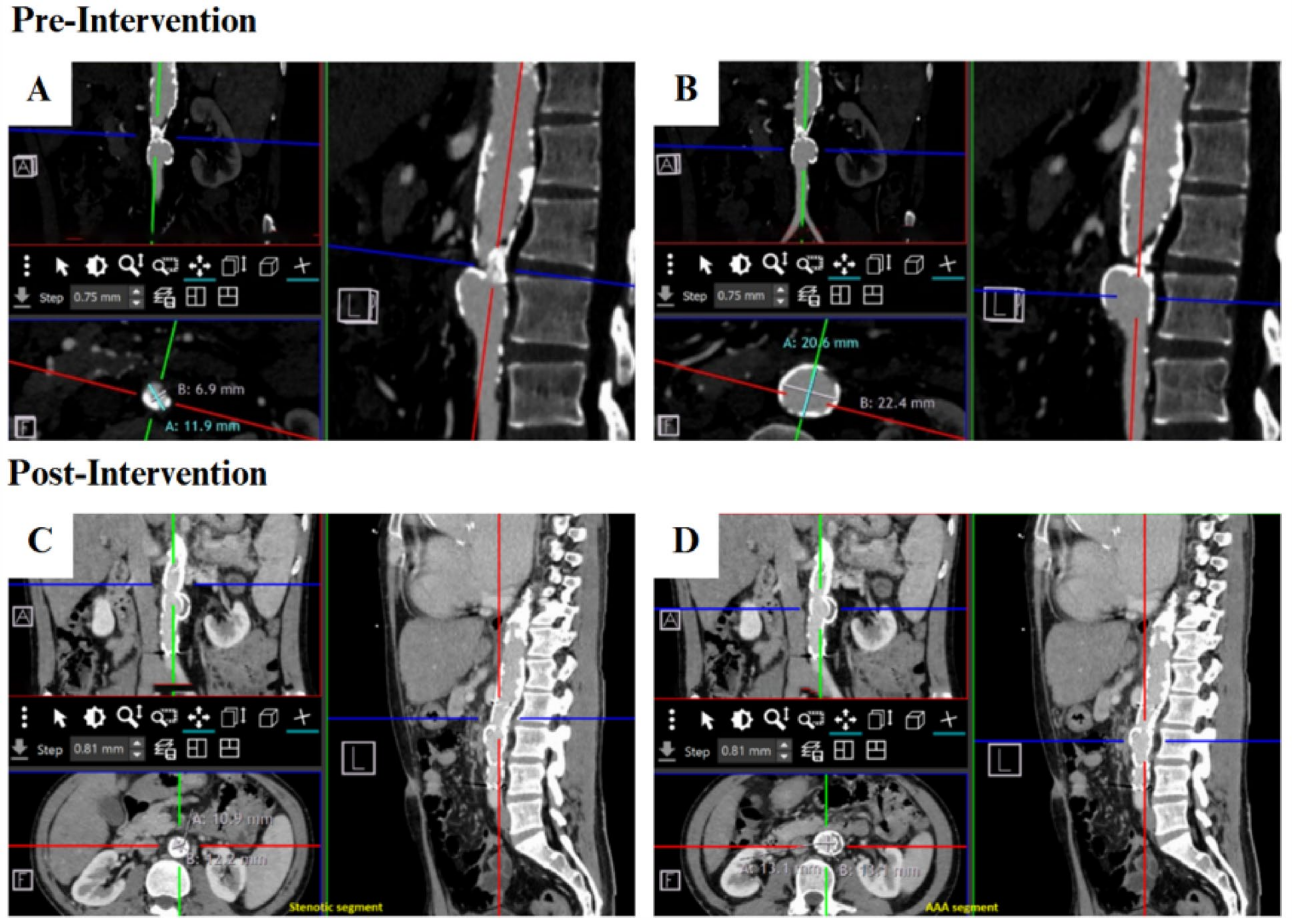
Six-month postoperative thoracoabdominal CTA. Post-endovascular repair, the stenotic infrarenal aorta improved from 6.9 × 11.9 mm to 10.9 × 12.2 mm. The saccular abdominal aortic aneurysm decreased from 20.6 × 22.4 mm to 13.1 × 13.1 mm
